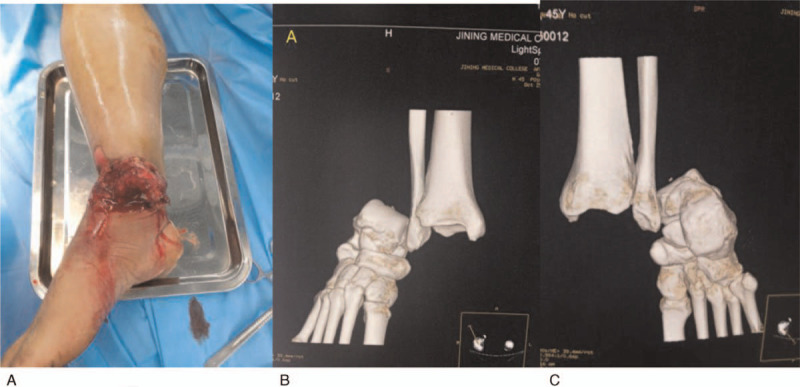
(A) The patient, a 45-year-old male, had an open wound with ankle bone and articular cartilage exposed; (B, C) Emergency three-dimensional CT examination of the ankle joint showed total ankle joint dislocation without fractures.
